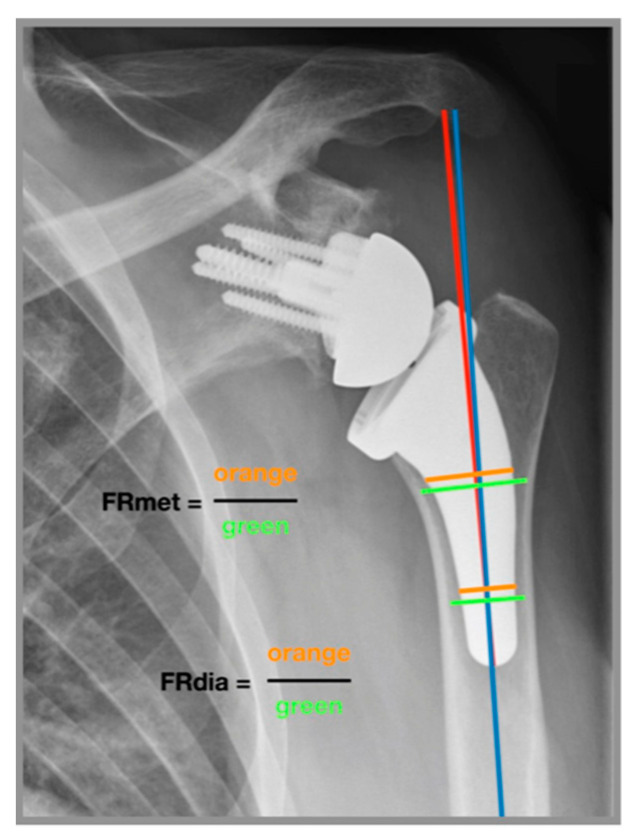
Measurement of filling ratio and alpha angle [27]. Anteroposterior radiograph of a left shoulder. The alpha angle (*α*) was measured between the shaft axis (blue line) and the stem axis (red line). Filling ration at the level of the metaphysis (FRmet) is a line perpendicular to the shaft axis, intersecting at the distal–medial border of the humeral platform. Filling ratio at the level of the diaphysis (FRdia) is a line perpendicular to the shaft axis, intersecting at the distal third of the prosthesis. The filling ratio is the quotient of the orange and green lines at the metaphysis (FRmet) and at the diaphysis (FRdia). Distances were calibrated based on the size of the glenosphere.
